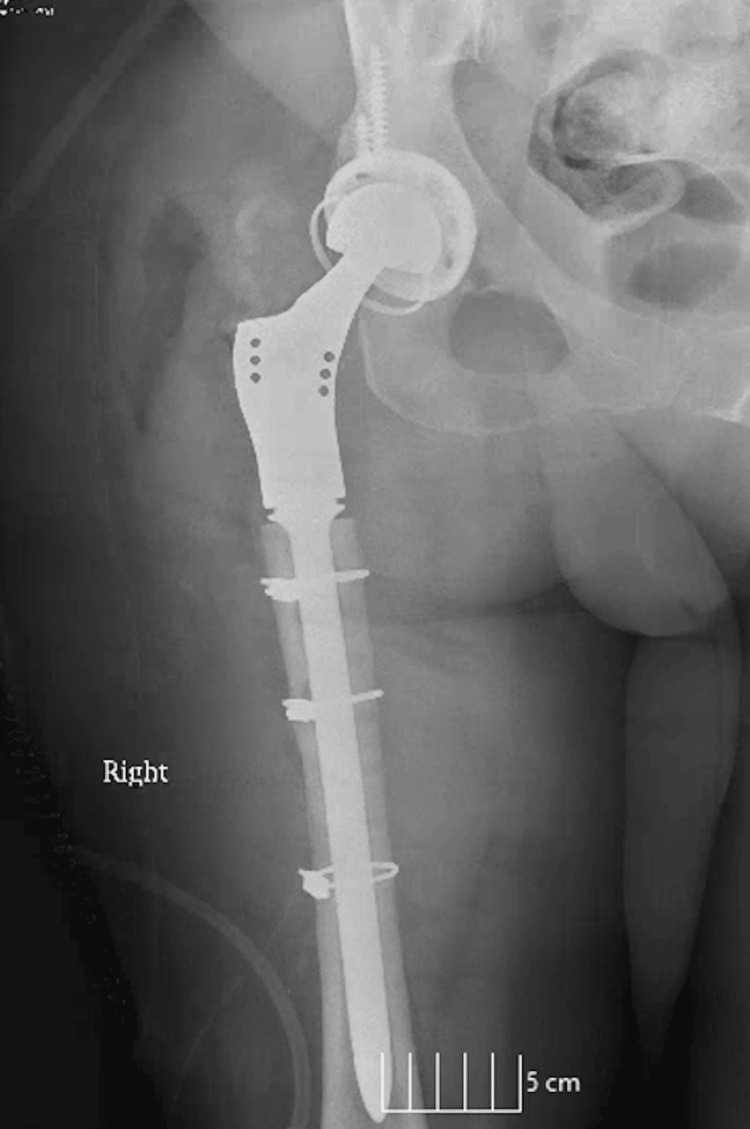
Postoperative X-ray - proximal femur replacement (PFR)
